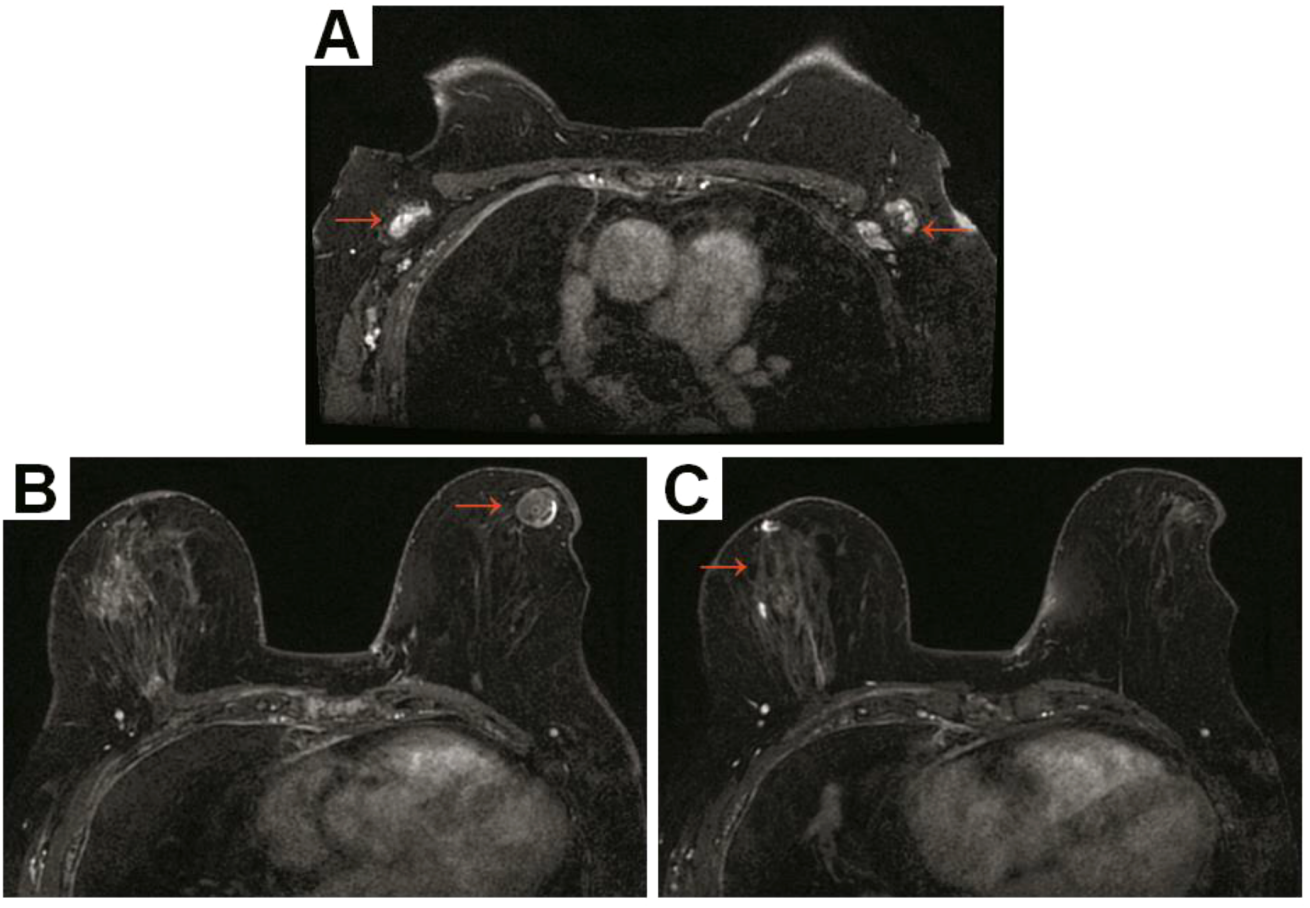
Breast magnetic resonance imaging revealed that bilateral axillary lymph nodes with enlargement and enhancement (A), an oval shape lesion at 6 o’clock on the left breast, with heterogeneous enhancement and no diffusion limitation (BI-RADS category 4) (B) and a focal area of non-mass enhancement at 6 o’clock on the right breast with heterogeneous enhancement, no diffusion limitation (BI-RADS category 4) (C).
